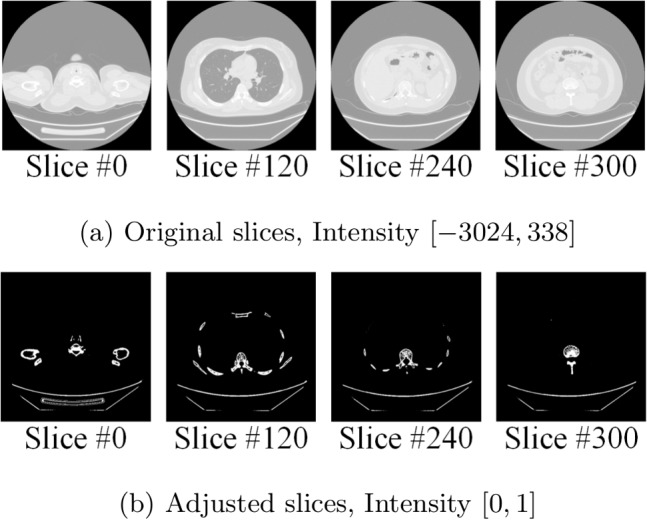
Intensity adjustment of chest CT slices. Adjusted slices show enhanced contrast for bone structures and effective removal of soft tissue components
